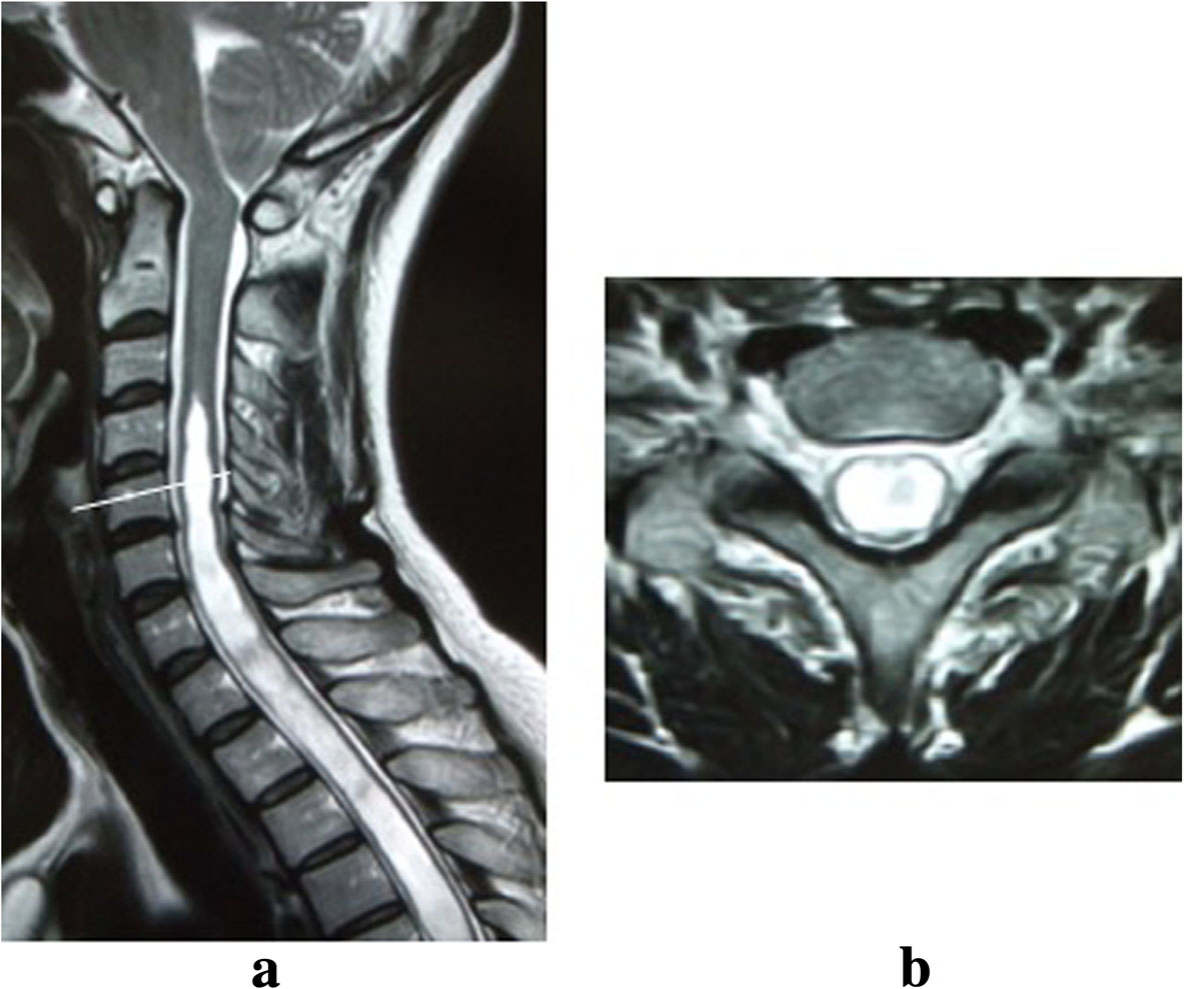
Preoperative cervical magnetic resonance imaging of a 54-year-old woman. a Sagittal image. The cerebellar tonsils are displaced below the level of the foramen magnum. There is a high T2-weighted signal observed in the cervical and thoracic cord. b Axial image at the C5 level. There is a high T2-weighted signal in the cervical cord
